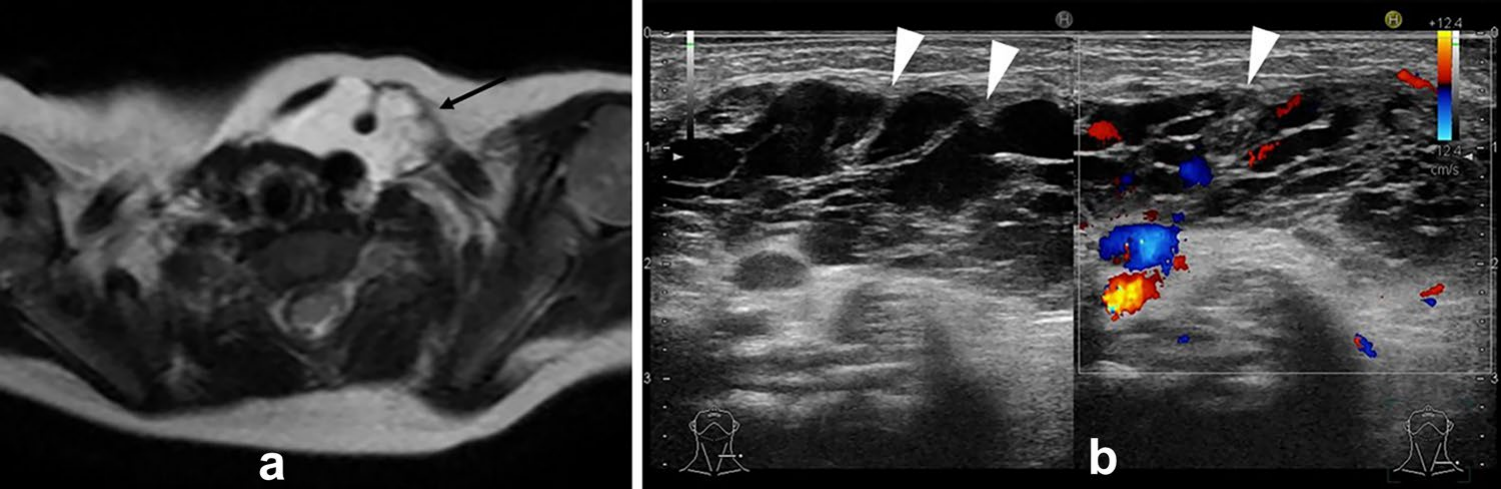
Three-month-old boy with lymphatic malformation. a T2 weighted image showed cystic lesion with high intensity (arrow) at left side of neck. b Ultrasonography showed cystic lesion with low echogenicity and some septa (arrow heads). The septa of this lesion were depicted clearer on ultrasonography than that of T2 weighted image
